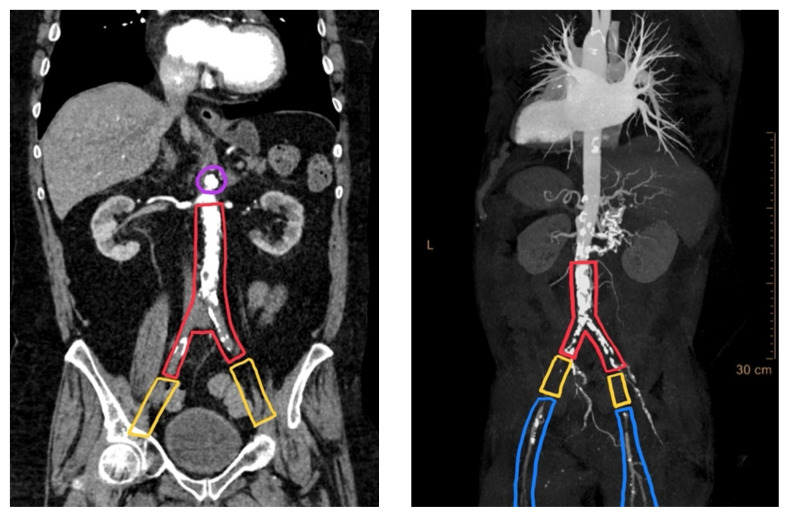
Annotated Axial CT Video Link: https://youtu.be/TYacFMkl-FM Unannotated Axial CT Video Link: https://youtu.be/MkgKogYWUnc
